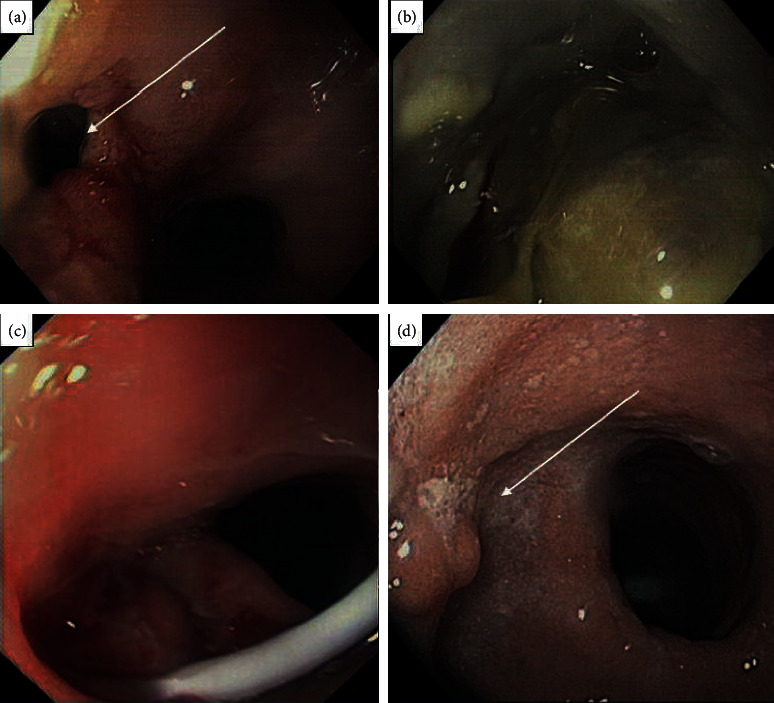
Endoscopic views of an early complicated patient. The GL documented with GST and CT scans now appears evident at endoscopic inspection ((a)) (with arrow). A large walled-off perifistular collection suspected at GST (Figure 1(b)) is confirmed at the endoscopic inspection (b). (c) EDPC positioned. (d) Endoscopic control at the 28^th^ POD: internal drainage acts like a foreign body and promotes the physiological re-epithelization of the leak (white arrow).
